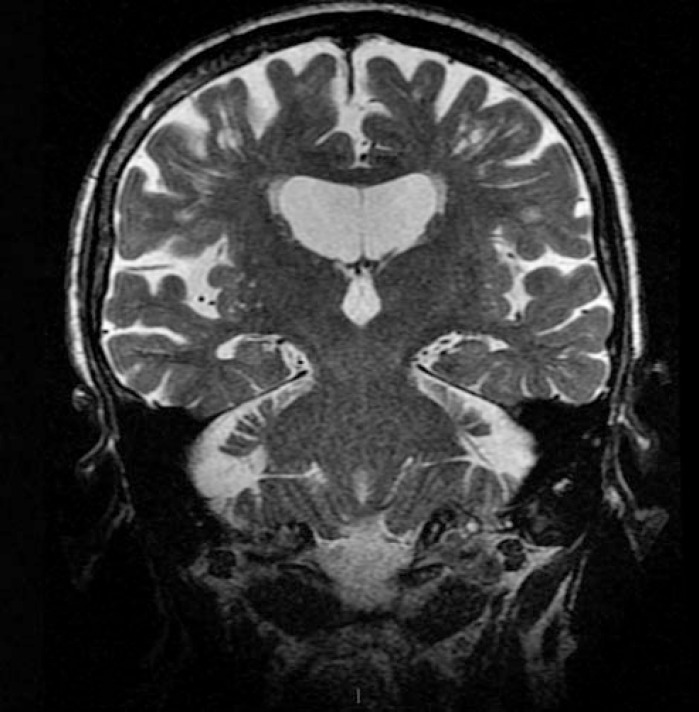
MRI with difuse cortical atrophy, frontal predominance.
